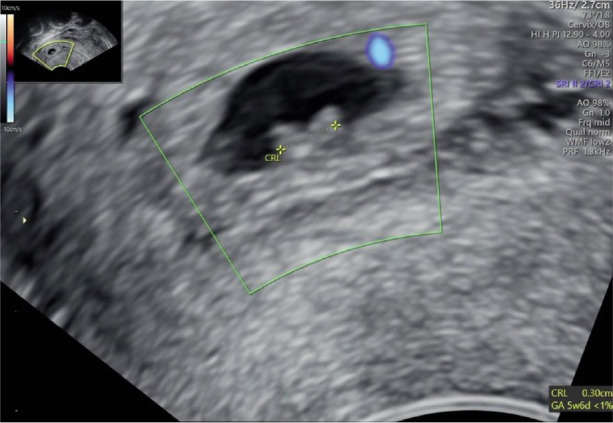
Ultrasound imaging on the 10th day presenting an embryonic pole with absence of heart activity.
